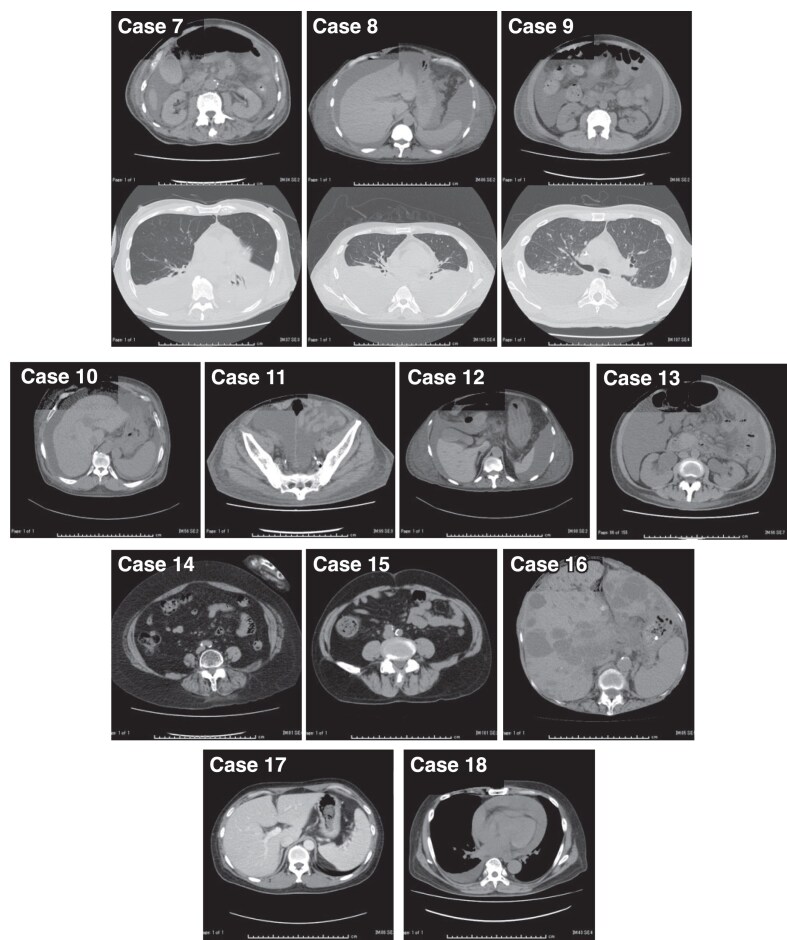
CT images of the patients with respiratory distress (patients 7–18). Patients 7–13 had massive ascites and pleural effusions. Patients 14 and 15 were obese. Patients 16 and 17 exhibited organomegaly and patient 18 had pericardial effusion.
